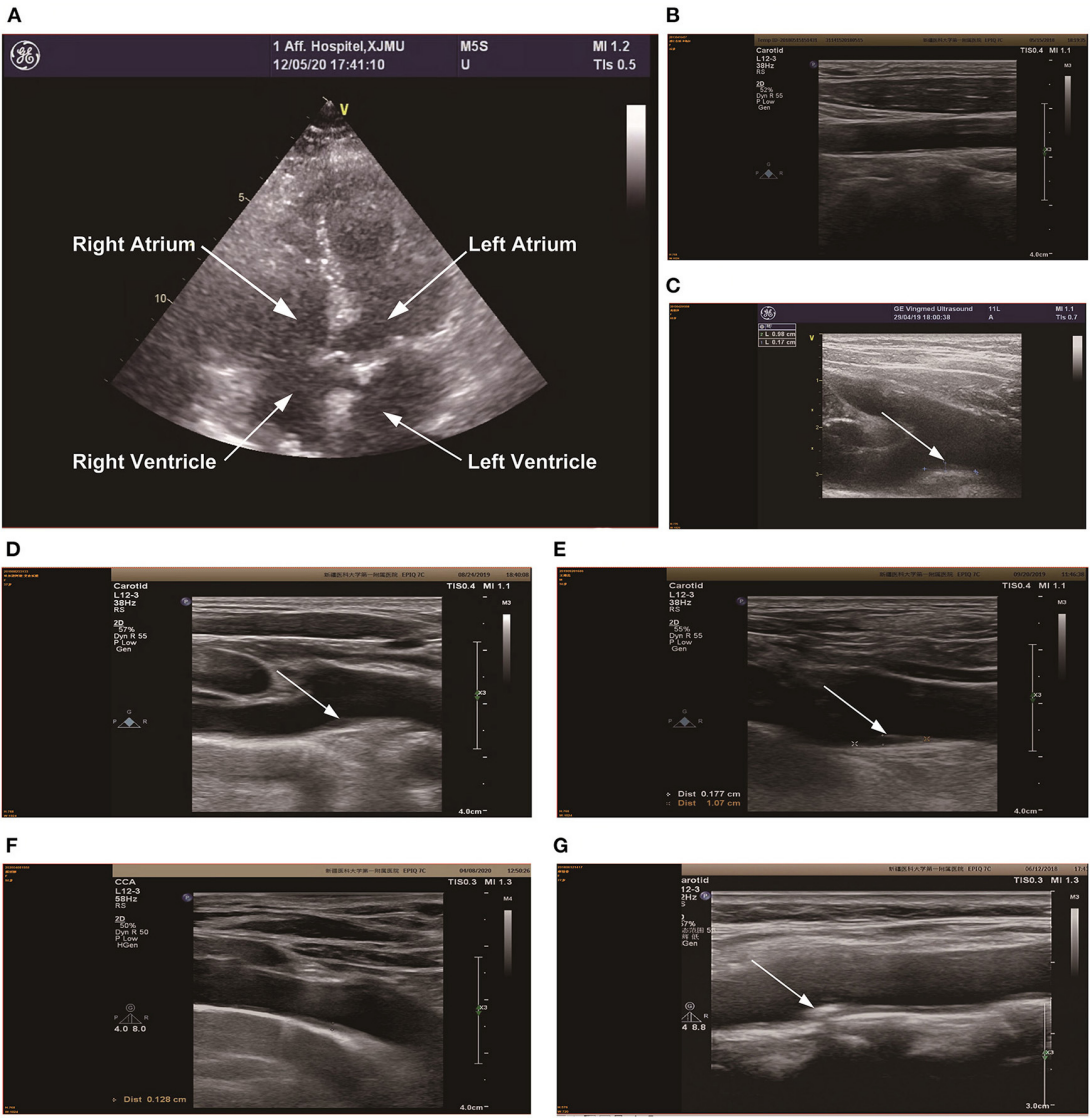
Color Doppler ultrasonography of the neck and heart was performed in 6 patients. (A) The heart color Doppler ultrasound image of patient 9, and the results suggested left atrial enlargement, reduced left ventricular myocardial phase motion, mitral valve insufficiency, active surface insufficiency, and mild pulmonary hypertension. (B) is the cervical vascular color Doppler ultrasound of patient 3, there were no obvious abnormalities in cervical vascular ultrasound. (C) is the cervical vascular color Doppler ultrasound of patient 4, and the results suggested that the innameless artery bifurcation and the plaque size of the right subclavian artery was about 9.8 × 1.7 mm, which has been marked in the figure. (D) is the cervical vascular color Doppler ultrasound of patient 5, and the results suggested that the innameless artery bifurcation and the plaque size of the right subclavian artery was about 10 × 1.8 mm, which has been marked in the figure. (E) is the cervical vascular color Doppler ultrasound of patient 6, and the results suggested that the innameless artery bifurcation and the plaque size of the right subclavian artery was about 11.1 × 1.8 mm, which has been marked in the figure. (F) is the cervical vascular color Doppler ultrasound of patient 8, intima thickening of the left carotid artery (1.2 mm), bifurcation of the innominate artery and thickening of the right subclavian artery (1.3 mm), which has been marked in the figure (G) is the cervical vascular color Doppler ultrasound of patient 9, there were no obvious abnormalities in cervical vascular ultrasound.
